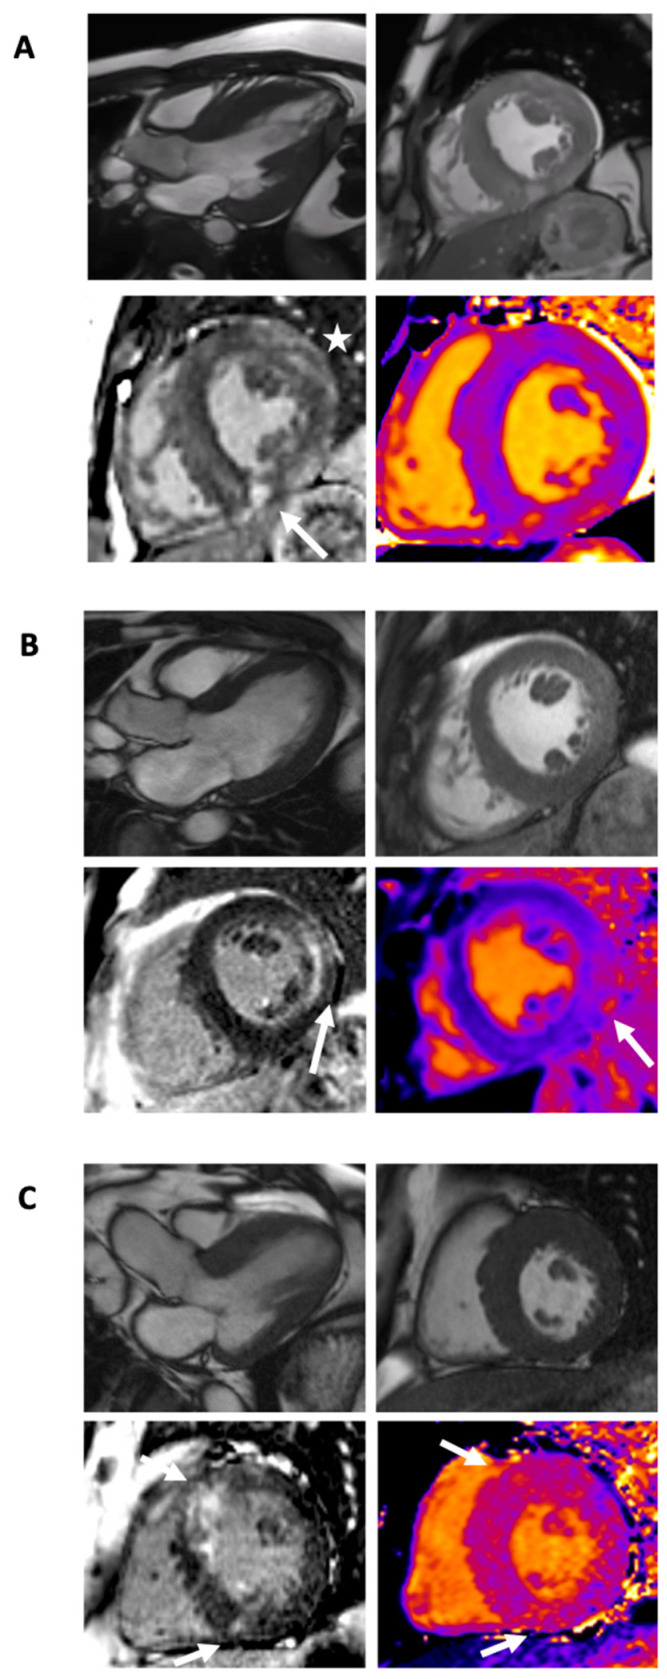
The differential diagnosis: cardiac MRI in left ventricular hypertrophy. Clockwise from left: still image from cine steady-state free precession three-chamber and mid-short-axis views, mid-short-axis T1 map, and mid-short-axis LGE image. Note how visually the three ventricles in Panels (A–C) look similar in morphology but have different tissue characteristics. (A) MELAS: There are moderate symmetrical left ventricular hypertrophy and normal right ventricular wall thickness. Native T1 mapping values are within normal limits with islands of higher values. LGE imaging identifies patchy mid-wall enhancement predominantly affecting the lateral wall (star), but also the septum with denser epicardial enhancement inferiorly (arrow). (B) Anderson–Fabry Disease: There is moderate symmetrical left ventricular hypertrophy with septal attenuation, with right ventricular hypertrophy. Native T1 mapping values are low (darker purple) in the septum, and normal in the inferolateral wall. This corresponds with LGE in the inferolateral wall (arrows). (C) Hypertrophic cardiomyopathy: There is asymmetrical hypertrophy affecting the basal to mid anterior wall and anteroseptum. Native T1 values are diffusely higher, with areas of relatively higher values (arrows) at both septal RV insertion points. LGE is seen in the septum and insertion points, in the area of maximal hypertrophy.
